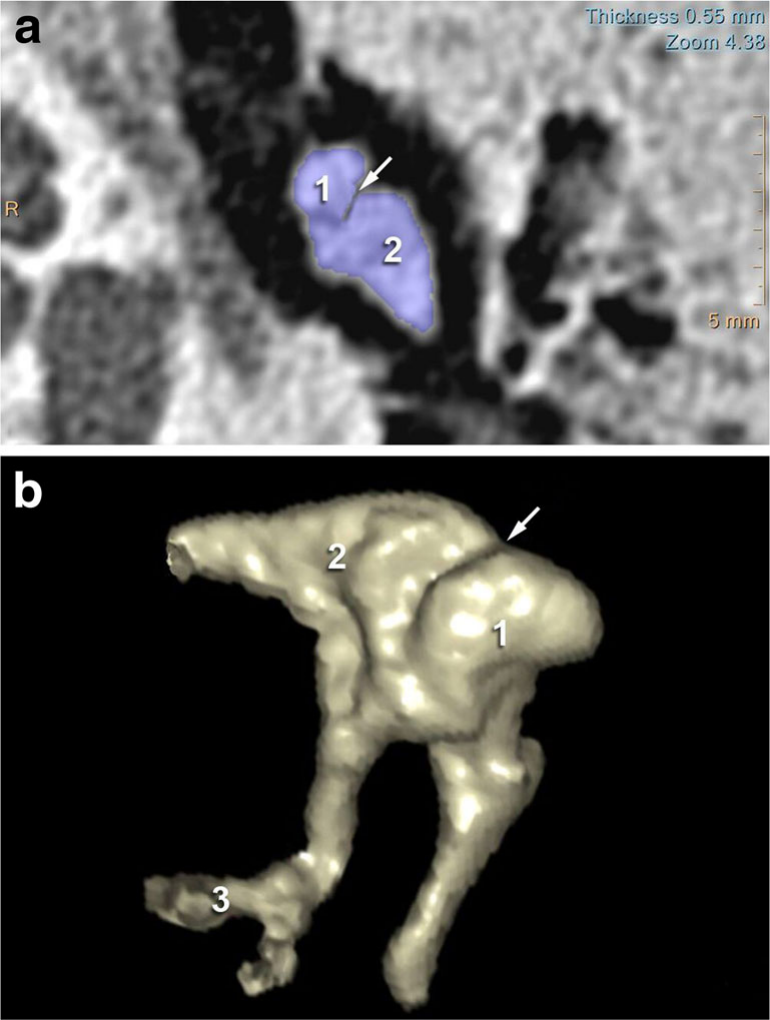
2-D CT images in the axial plane showing a normal incudo-malleolar joint. The ice-cream cone including the body of the incus (2) and the head of the malleus (1) 3-D CT reveals that malleus (1) and incus (2) are in close contact in the healthy middle ear
